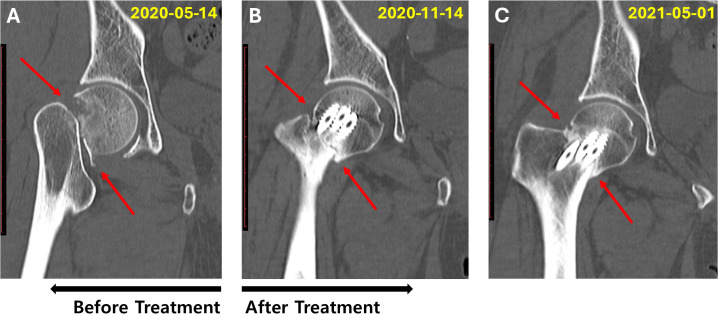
Computed tomography images of the right hip joint of Case 3, a 58-year-old woman with a femoral neck fracture. (A) Frontal planes of the right hip joint, showing the fractured femoral neck immediately after the accident and before surgery (May 14, 2020); (B) Frontal planes of the right hip joint 6 months after the fracture and 1 month after the diagnosis of delayed union (1-month follow-up after JGT administration) (November 14, 2020); (C) Frontal planes showing distinct changes in the right hip joint, demonstrating femoral neck fracture healing (May 1, 2021).
